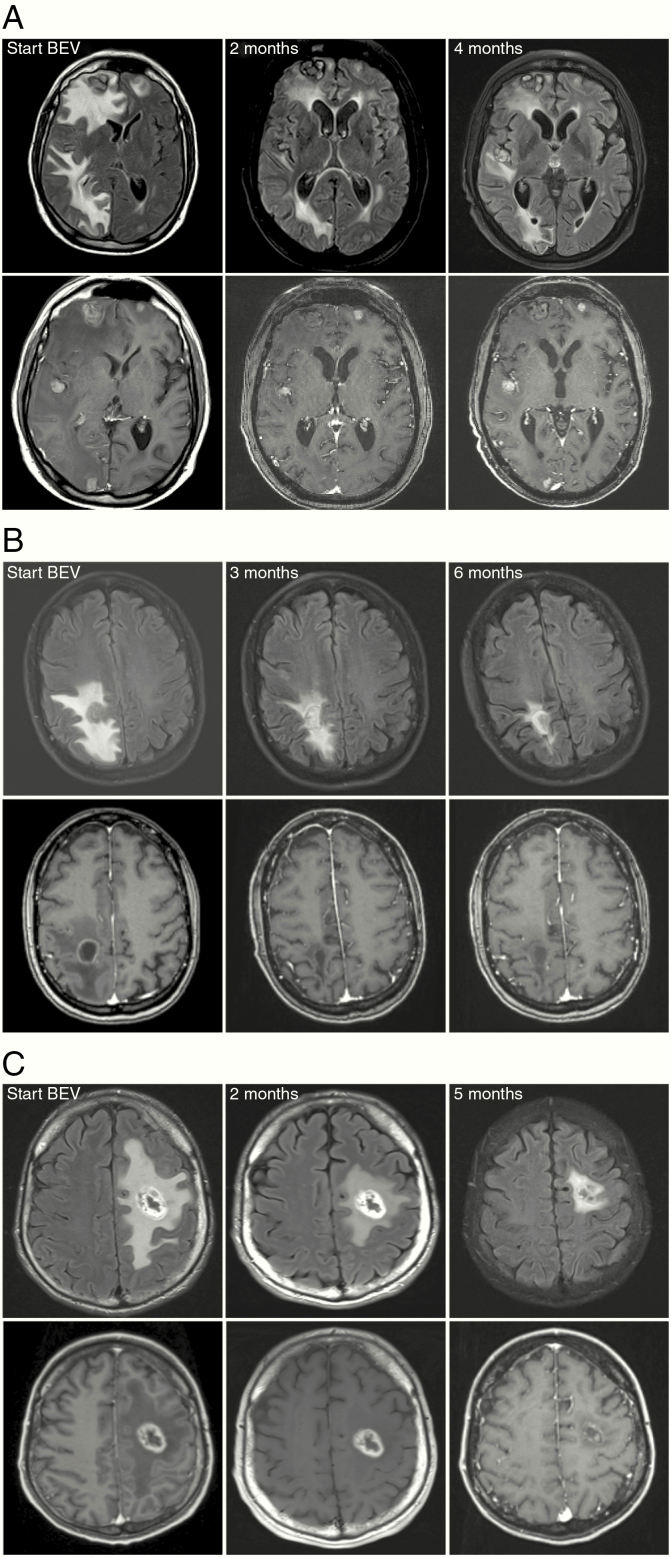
(A) MR images of baseline (BL) and follow-up MRI of a lung cancer patient with a partial response after initiation of bevacizumab-based treatment. Partial response is seen both on FLAIR (upper row) and T1-weighted (lower row) images. (B and C) MR imaging examples of patients with breast cancer (B) and colorectal cancer (C) showing partial response on FLAIR and complete response on T1-weighted images after Gd-contrast administration (B) on follow-up MRI. Note that the tumor volume (central core) and perifocal edema can be differentiated on FLAIR images.
